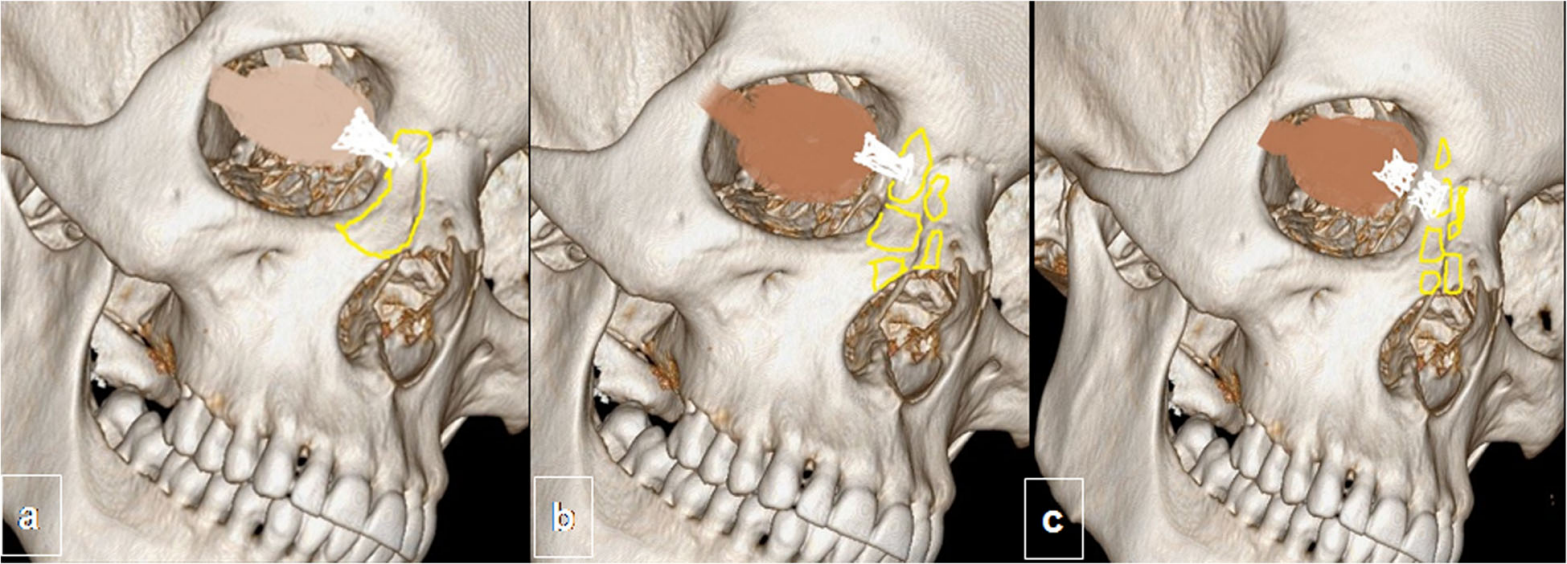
Classification of naso-orbito-ethmoidal fractures. Three dimensional CT images of an adult skull with color overlays depict the Markovitz and Manson classification: type I (a) characterized by a single large fragment with attached medial tendon; type II (b) with bone comminution without extension to the medial canthal tendon; and type III (c), where comminution affects the medial canthal tendon
